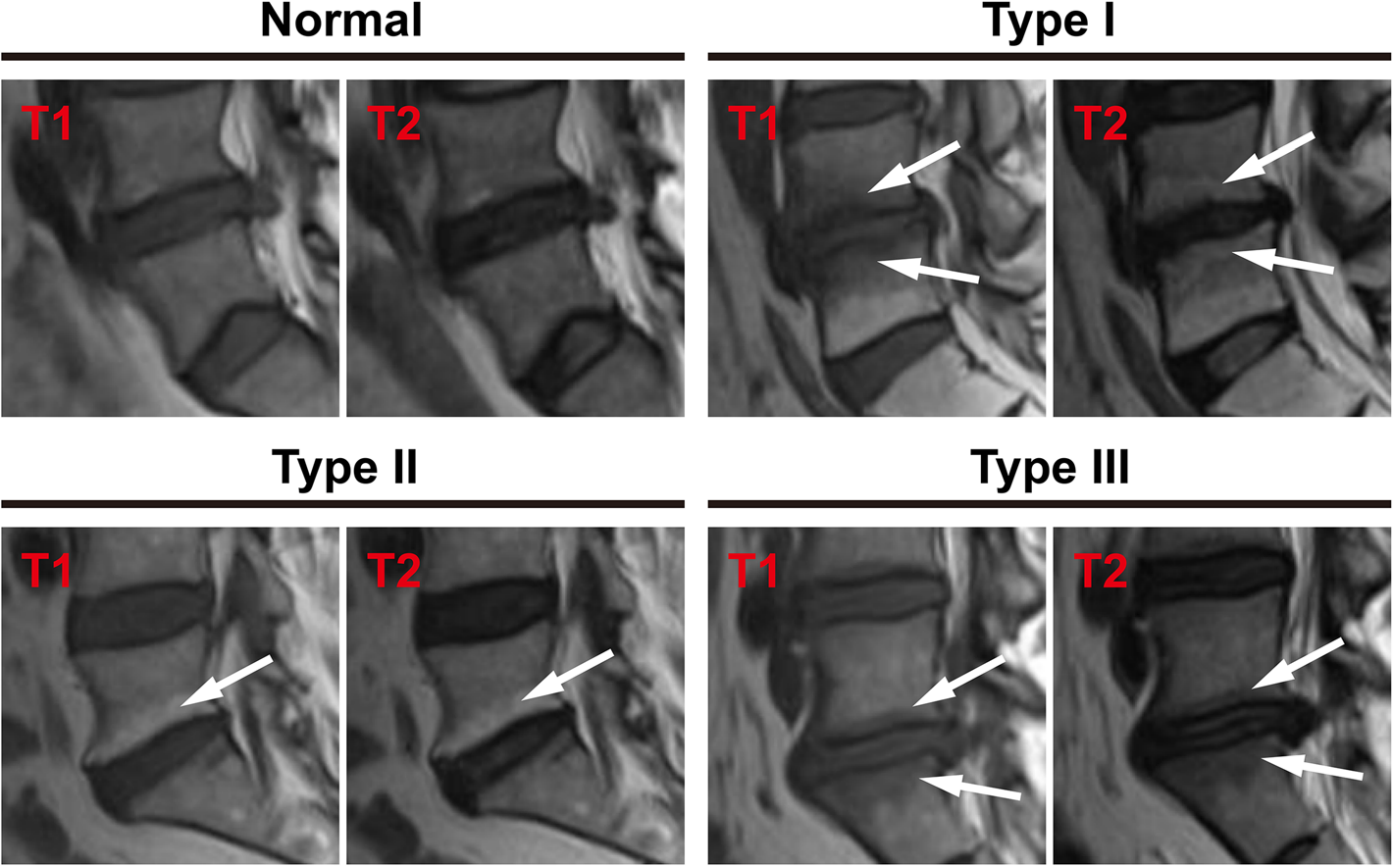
The classification of Modic change. On T1-weighted and T2-weighted images, normal: isointense on T1w and T2w. Type I, hypointense on T1w and hyperintense on T2w. Type II, hyperintense on T1w and hyperintense or isointense on T2w. Type III, hypointense on T1w and T2w
